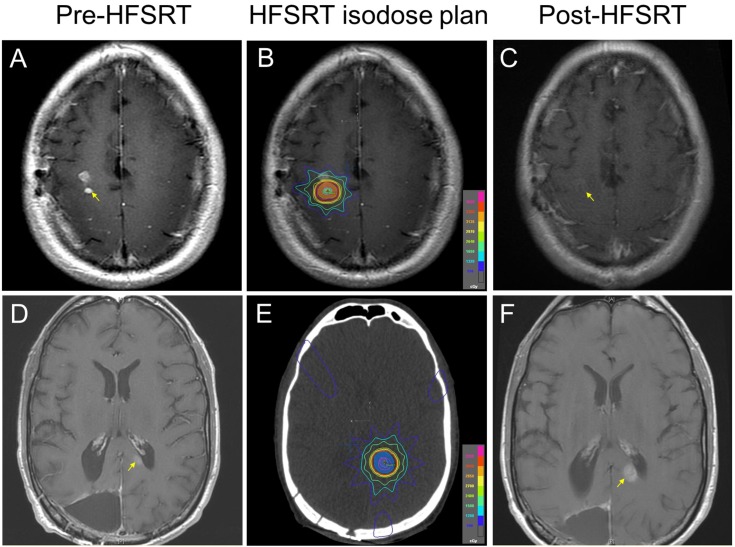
Representative images of MRI prior to and after therapy and radiation treatment plans of patients’ recurrent GBM tumors treated with stereotactic radiation (A) A right contrast-enhancing lesion in the superior centrum semiovale, visualized on a T1 contrast pre-treatment MRI scan (arrow). (B) A stereotactic radiation plan demonstrating isodose lines covering the target lesion. (C) A follow-up axial T1 contrast MRI scan demonstrating a resolution of the tumor on the at 22 months post-treatment (arrow). (D) A left contrast-enhancing lesion in the major forceps of the corpus callosum visualized on a T1 contrast pre-treatment MRI scan (arrow). (E) A stereotactic radiation plan demonstrating isodose lines covering the target lesion. (F) A follow-up axial T1 contrast MRI scan demonstrating tumor progression at 2.2 months post-treatment (arrow).
